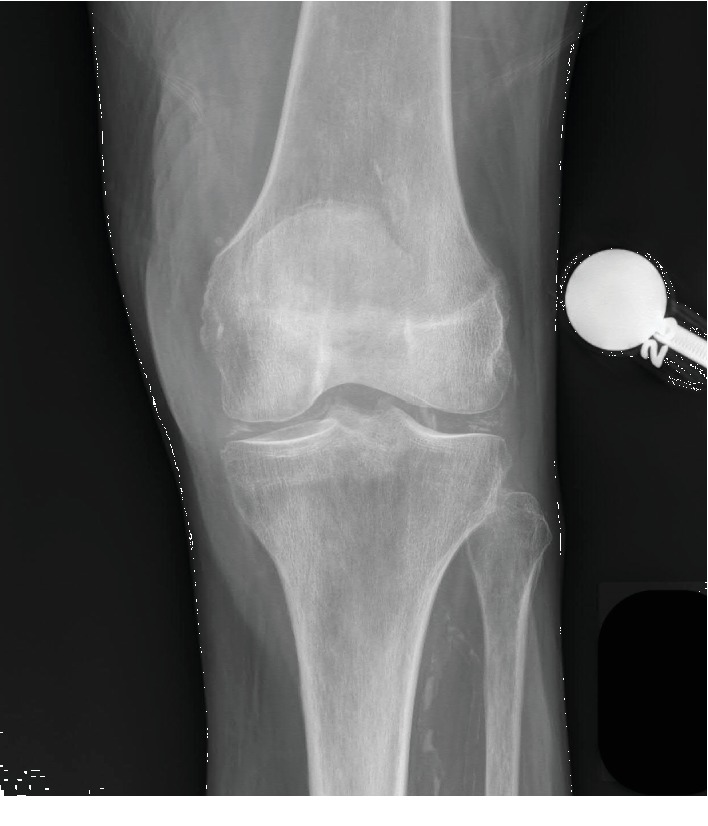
Anteroposterior radiograph of the left knee demonstrating chondrocalcinosis.
